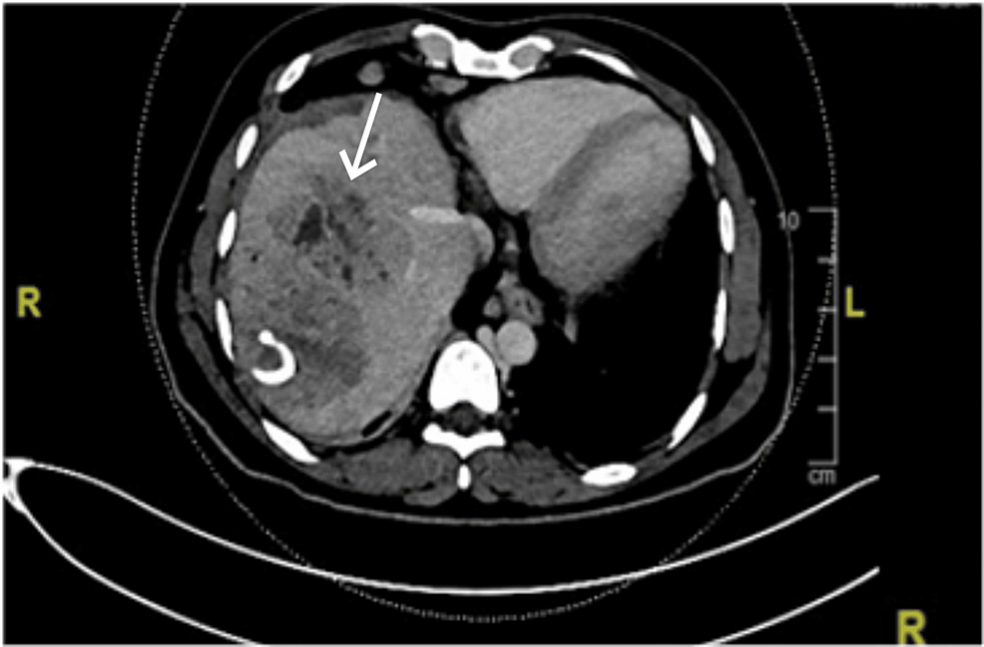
Axial computed tomography scan of the abdomen and pelvis showing an increase in the size of the previous abscess, with new abscess formation (3.7 x 4.6 x 6 cm), focal intrahepatic ductal dilation, and atrophic left liver lobe.
